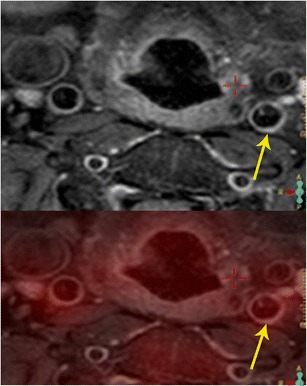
Carotid atherosclerosis. a Axial proton density-weighted MRI image (top) shows mild thickening of the wall of the left carotid artery (arrow) due to a plaque. b Axial fused PET/MR image at the same level shows uptake in the same area (arrow), indicative of plaque inflammation
